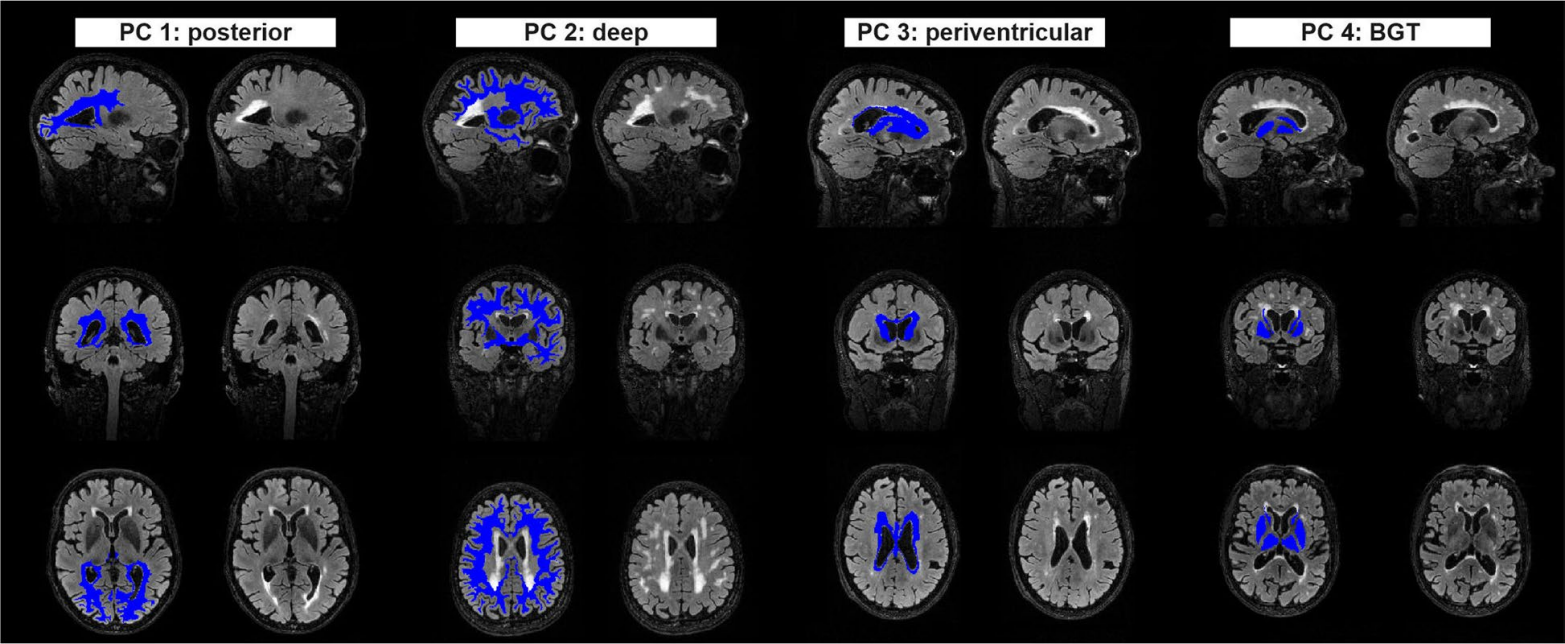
FLAIR images of participants exhibiting the highest principal component scores for each component, with and without overlaid bullseye parcels. Only parcels with loadings > 0.4 are indicated in the figure
